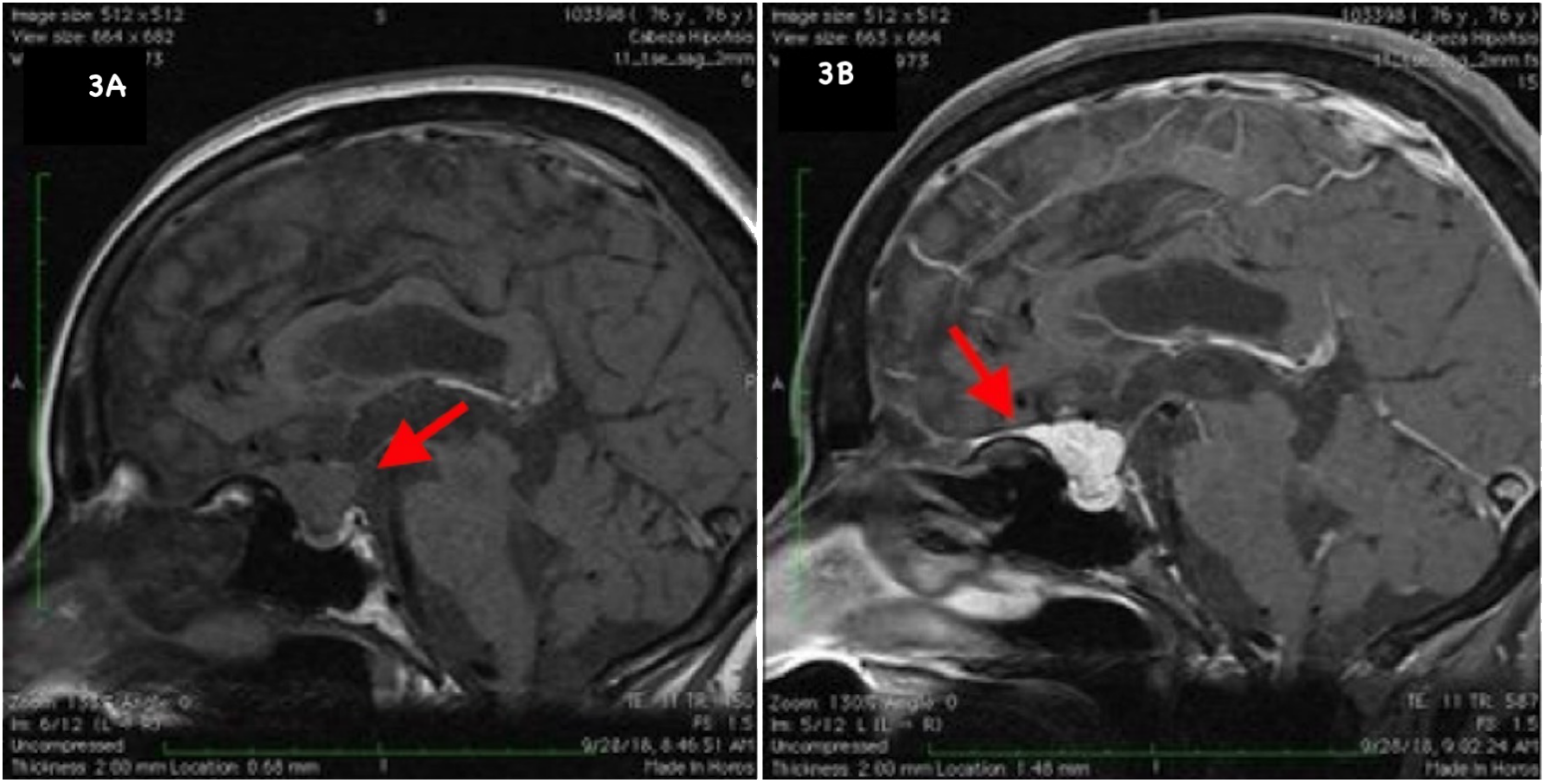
Sellar MRI. Sagittal (A): T1 pre-contrast shows an isointense suprasellar expansive process with intrasellar extension. (B): Hyperintense lesion after gadolinium administration. Dural tail sign, characteristic of sellar meningiomas.
